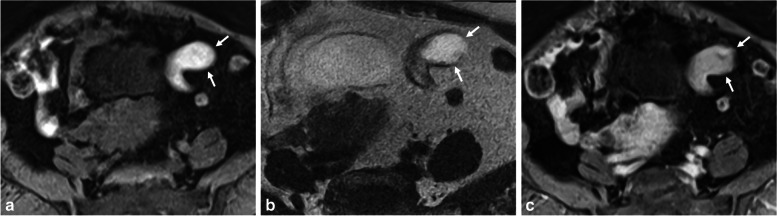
Chronic hydrosalpinx in a 58-year-old woman with pelvic pain and history of PID. a Axial T1-W fat-suppressed, (b) axial T2-W, and (c) axial T1-W fat-suppressed contrast-enhanced MR images show a fluid-filled fallopian tube (arrows) with high T1- and T2-signal intensity. Note the absence of fat stranding, peritonitis, or significant adnexal enhancement
